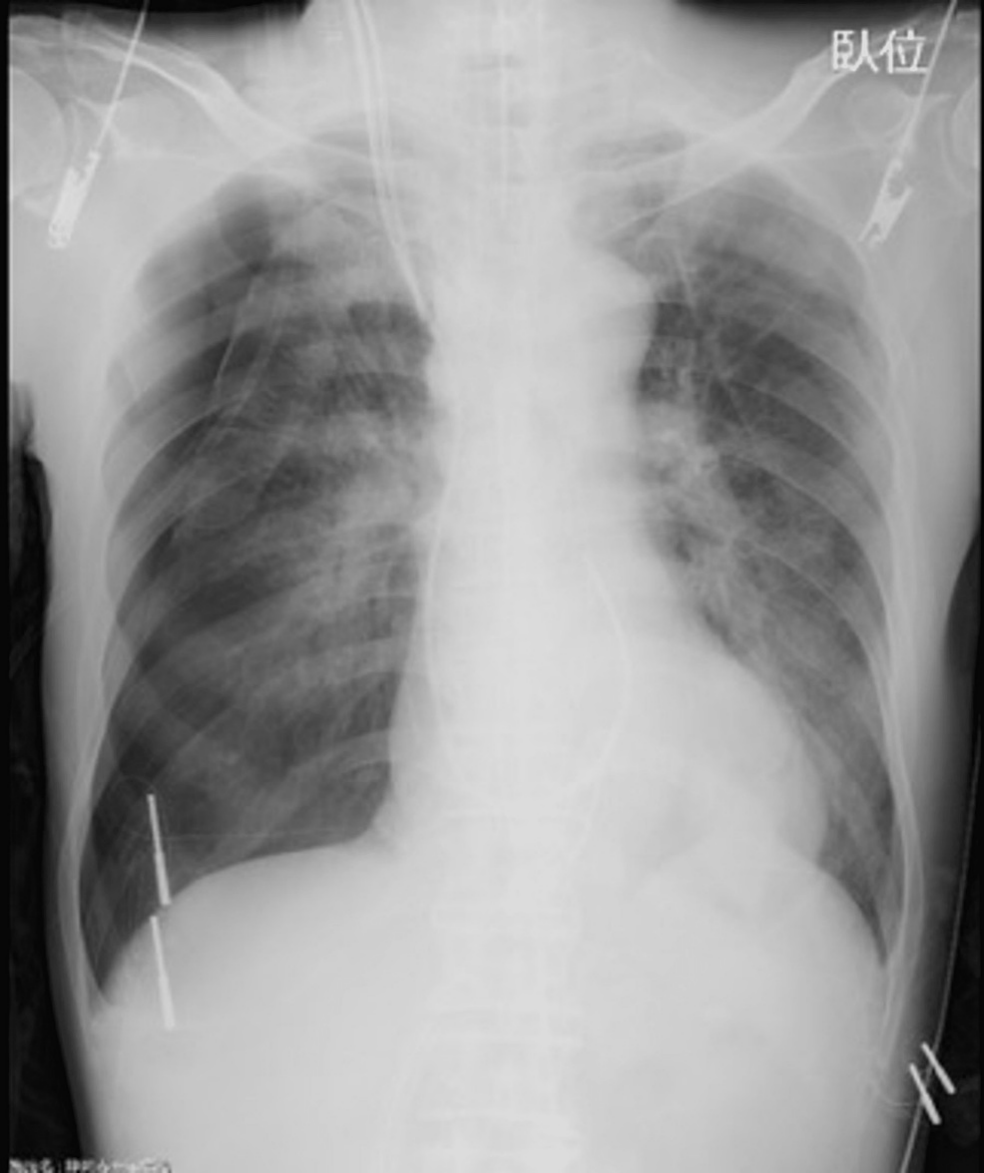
Chest radiograph on the first postoperative day. Right pneumothorax with a cardiothoracic ratio of 50.5% was revealed.
